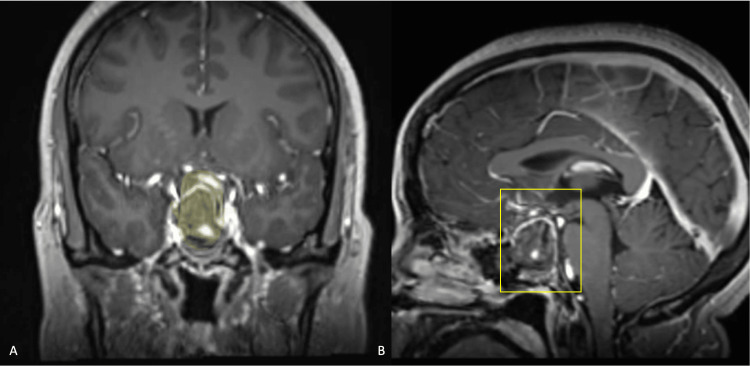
Postoperative C+ MRI in coronal (A) and sagittal (B) projections showing no residual tumor (previous tumor size is shown in yellow shading for comparison)
